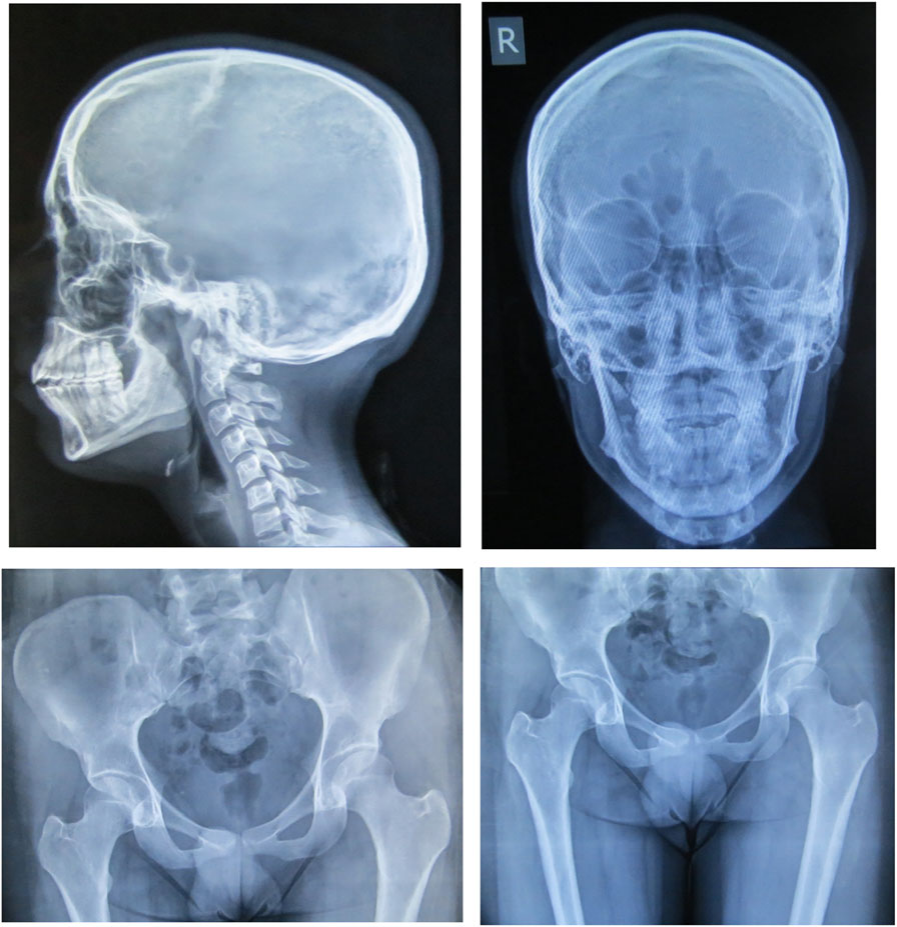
Bone survey of skull, hip, and femur. X-ray finding was normal
